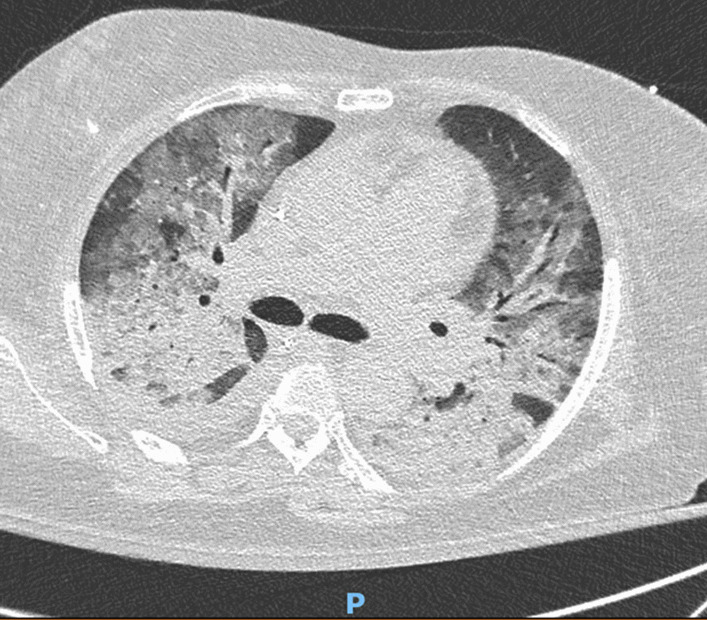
Lung computed tomography on ICU admission, with diffuse ground-glass opacities and septal thickening
